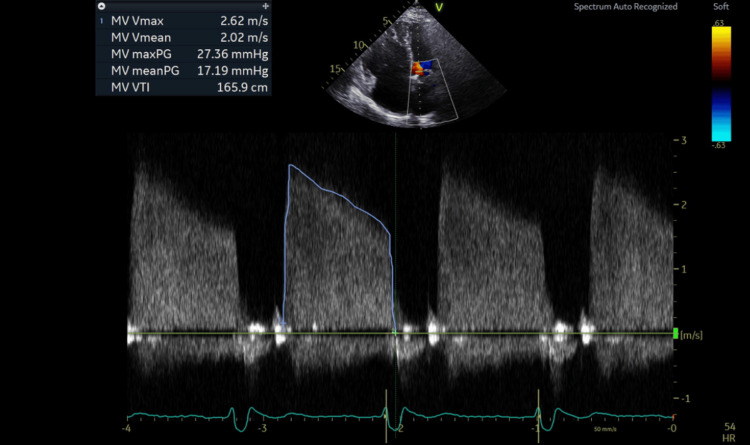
Spectral Doppler waveform prior to mitral valve in valve replacement. Mean pressure gradient across the mitral valve is 17.19 mmHg indicating severe mitral stenosis.
